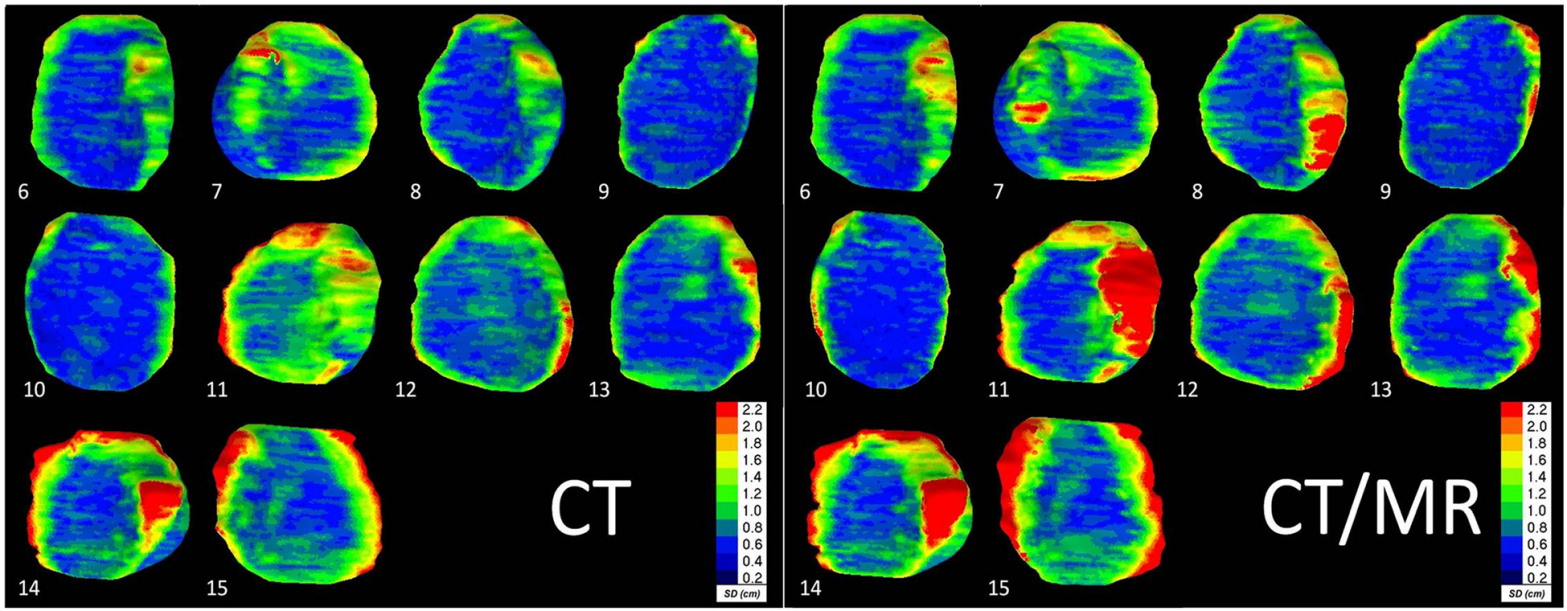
Left: Coronal posterior view of the ten delineated Clinical Target Volume (CTV) breast Computed Tomography (CT) volumes. Right: Coronal view of the ten delineated CTV breast CTMR volumes. The local surface distance variation of the four observers is projected on the median surface of each CTV breast. Colour map: Blue: high agreement between observers; Red: low agreement between observers according to the scale given.
